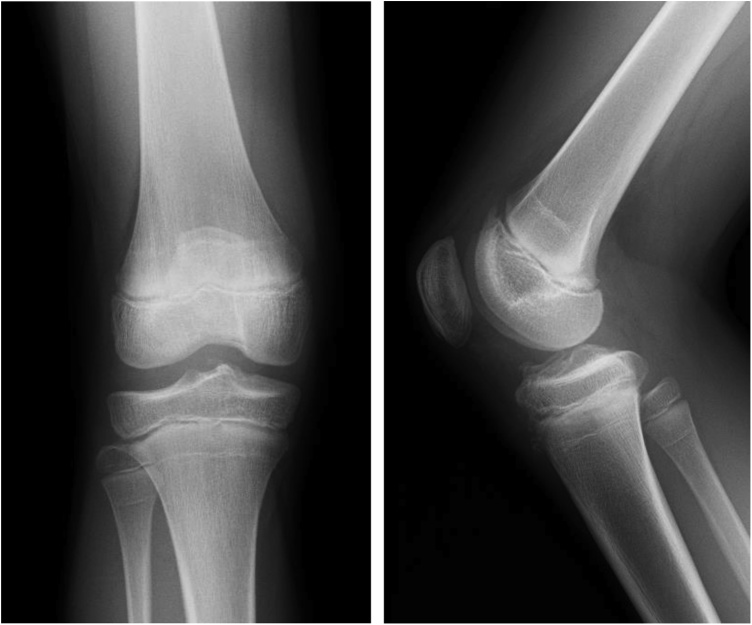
Radiographs showed bone healing and no dislocation of the bone fragment in the knee at the 1-year follow-up.
